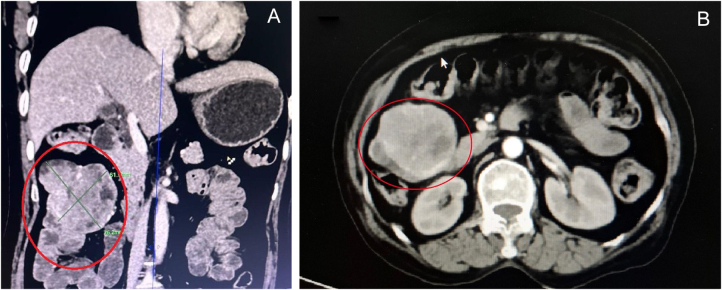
Contrast-enhanced computed tomography depicting a heterogeneously enhancing mass in upper right quadrant of abdomen (shown inside red ellipse). (A) Coronal section, (B) Axial section.
